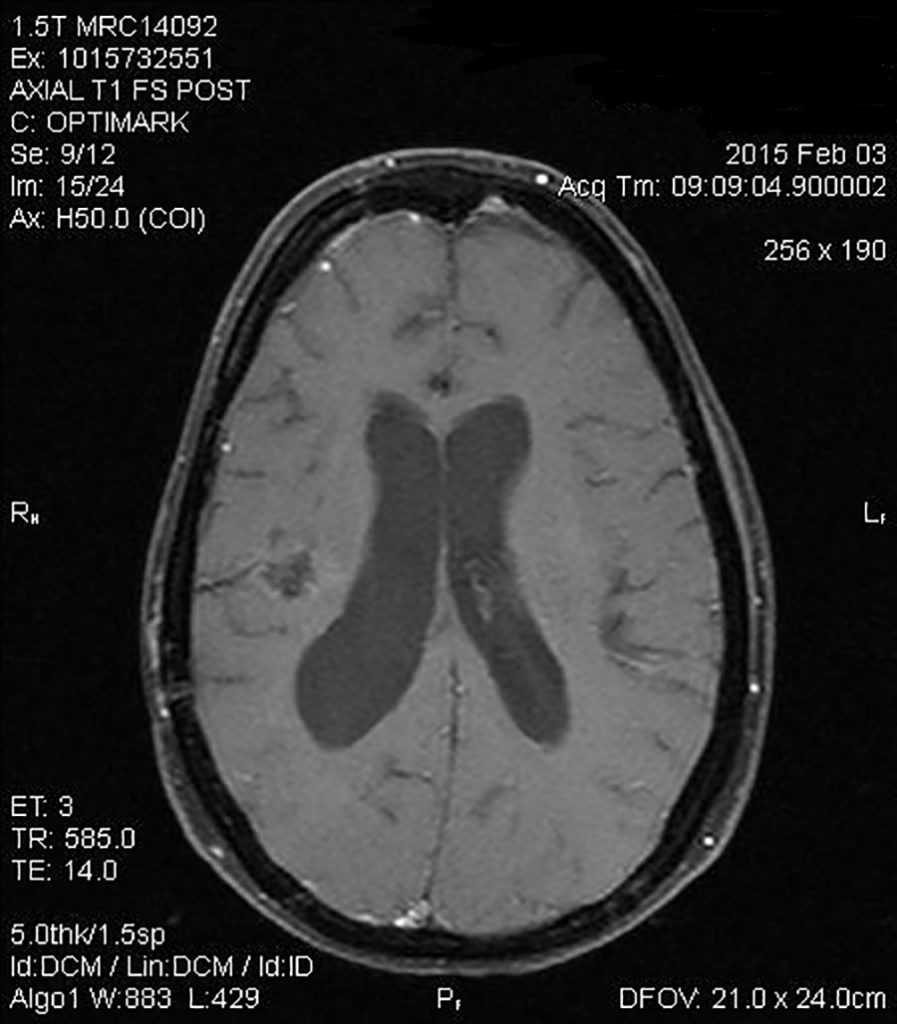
Post-treatment MRI of the brain with and without contrast. Postoperative changes in the right frontotemporoparietal region are shown. Irregular enhancement at the surgical site extends to the walls of the right lateral ventricle. This was unchanged in subsequent MRI scans.
